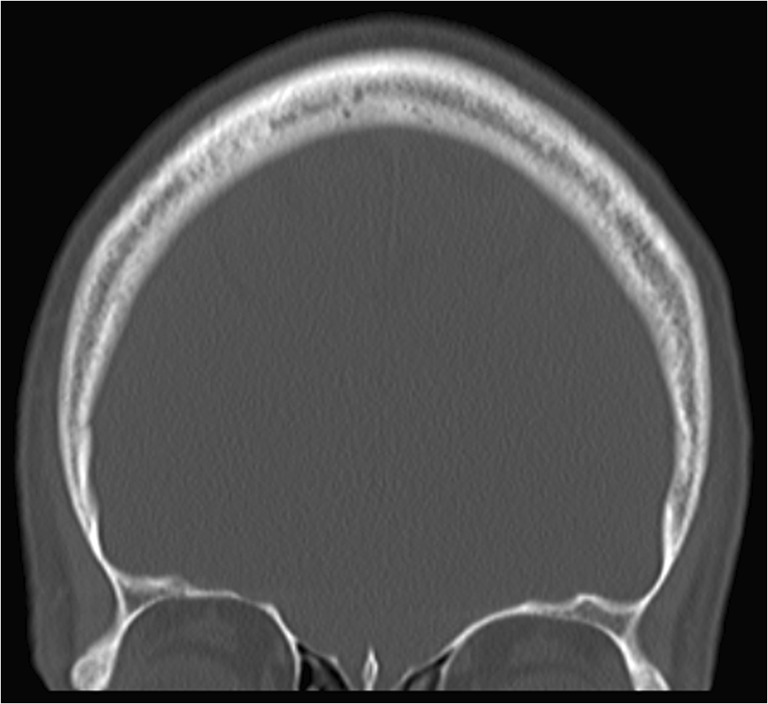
Field of view often seen in computed tomography imaging taken to exclude brain injury following head trauma
